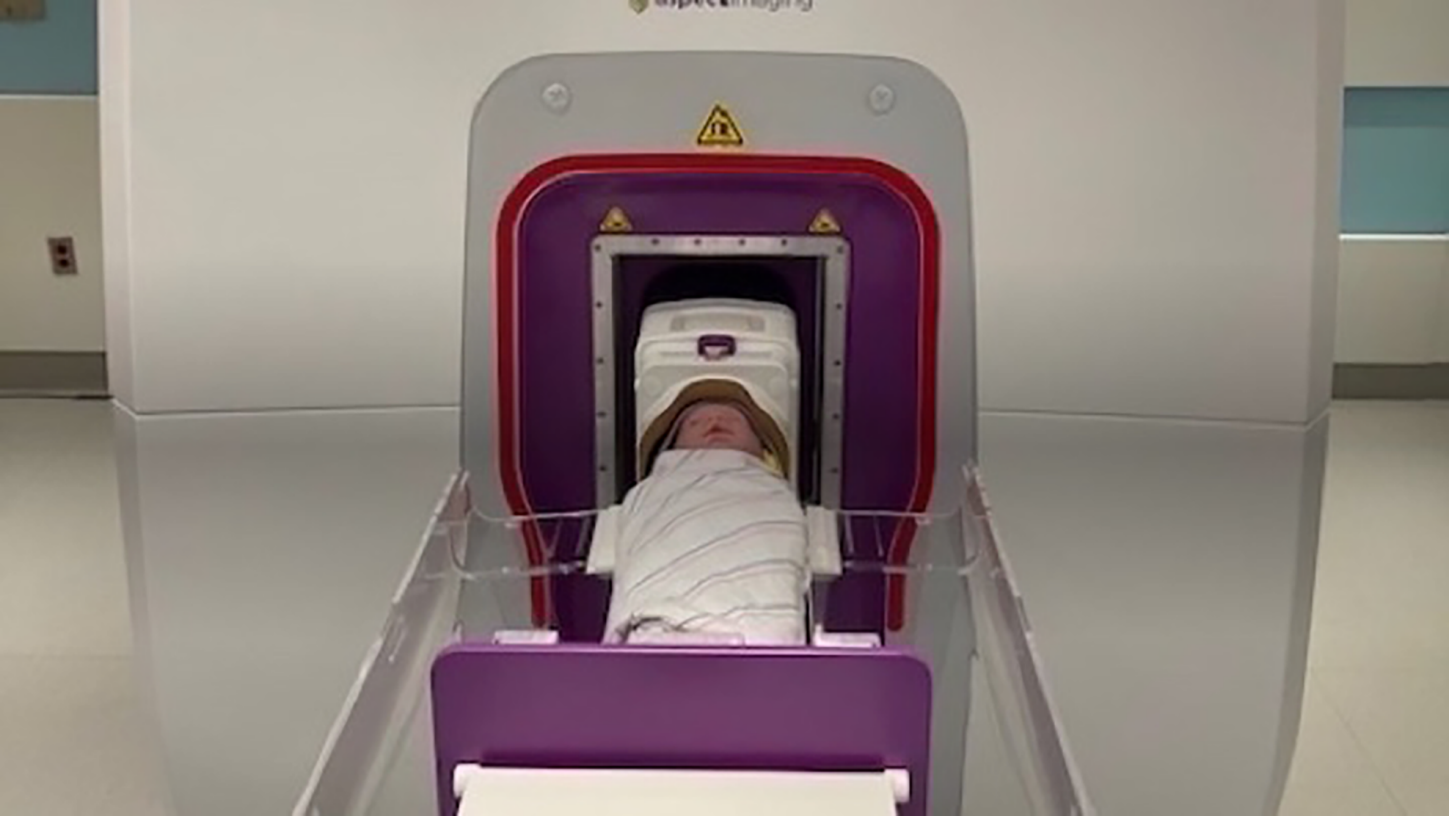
Procedure for brain MRI: infant wrapped in MRI-safe jacket is placed in Embrace Aspect scanner.
